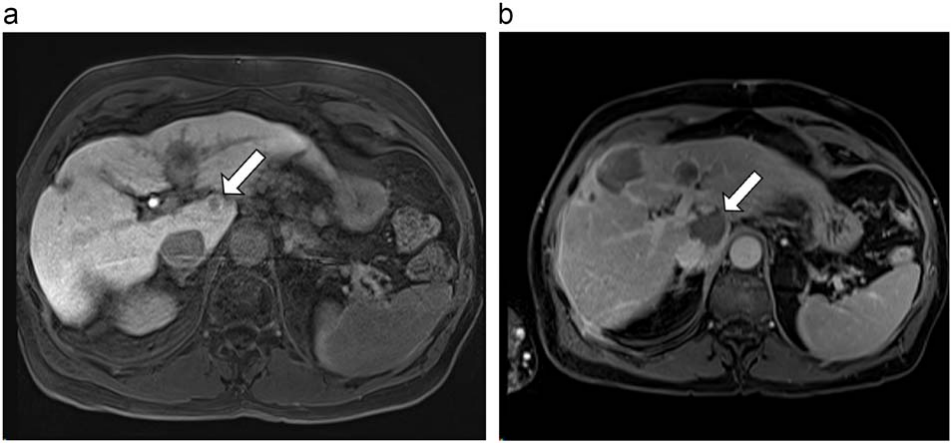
(a) Pre-ablation MRI demonstrating 10 mm caudate lesion. (b) Post-ablation MRI (14 days postoperatively) demonstrating 35.9 mm complete ablation of caudate lesion.
